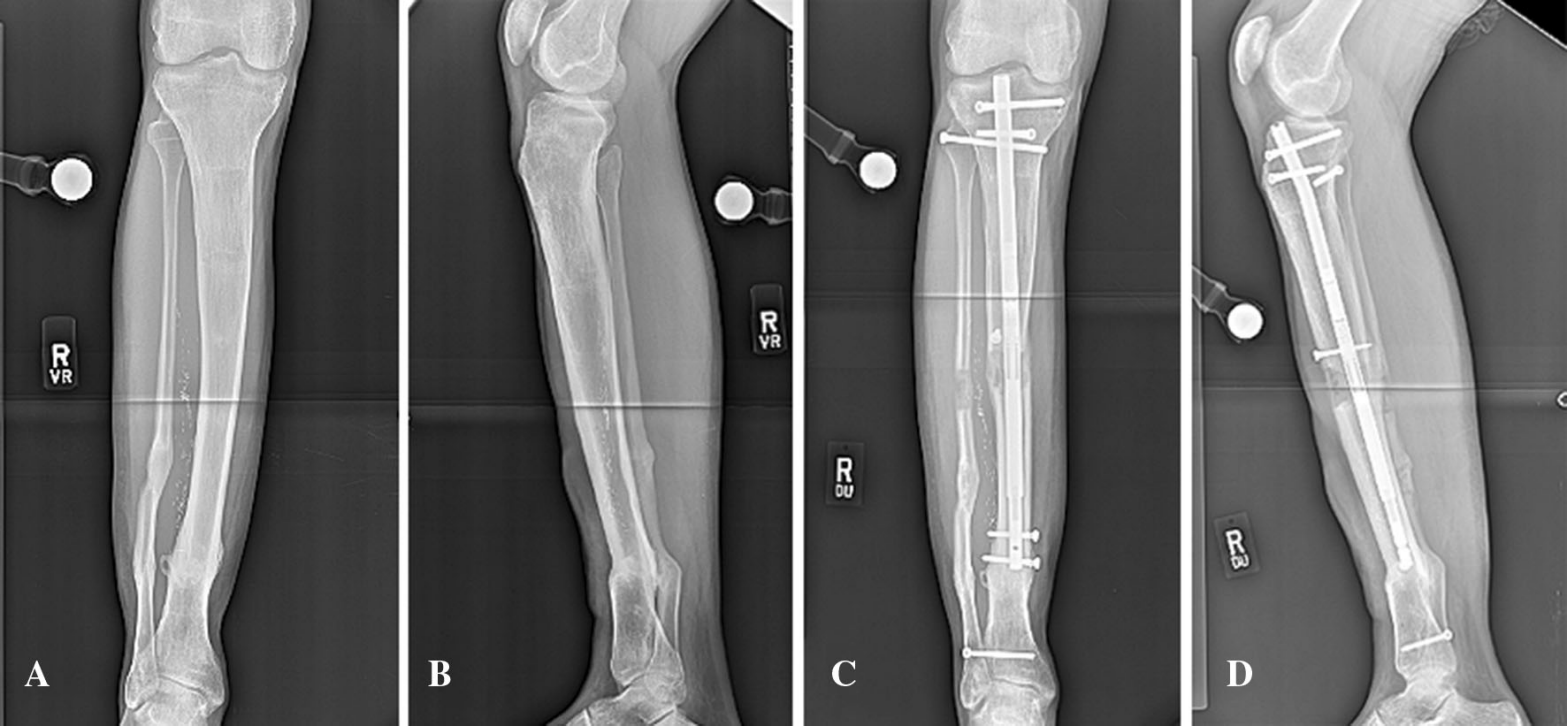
Antegrade tibial nail with mid-tibial osteotomy. Preoperative anteroposterior (a) and lateral (b) radiographs show preexisting valgus deformity of mid tibia. One lateral blocking screw was inserted near the osteotomy site in the proximal fragment to avoid valgus deformity during lengthening. Also, a blocking screw was inserted posterior to the nail in the proximal fragment before the reaming to guide the nail. Radiographs (c, d) represent post operative anteroposterior and lateral views at the end of distraction, respectively
